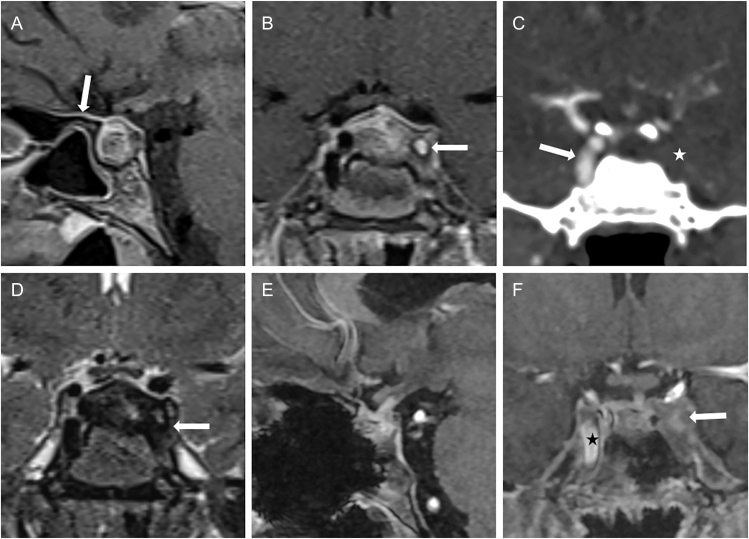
Case 3. (A) Sagittal T1-weighted MRI post-contrast at initial presentation shows an intrasellar space-occupying lesion. Strong dural enhancement is found (arrow). (B) Coronal T1-weighted MRI post-contrast shows occlusion of the left ICA (white arrow). (C) CT angiography confirms complete occlusion of the left ICA in the cavernous segment (asterisk) and a perfused right ICA with normal calibre (arrow). (D) T2-weighted MRI demonstrates parasellar T2 dark sign (arrow). (E) Sagittal T1-weighted MRI post-contrast shows reduced size of the lesion following surgery and GC therapy. (F) Coronal T1-weighted MRI post-contrast shows persistent occlusion of the left internal carotid artery following surgery and GC therapy (white arrow) and a regular flow signal in the normal-sized right internal carotid artery (black asterisk).
